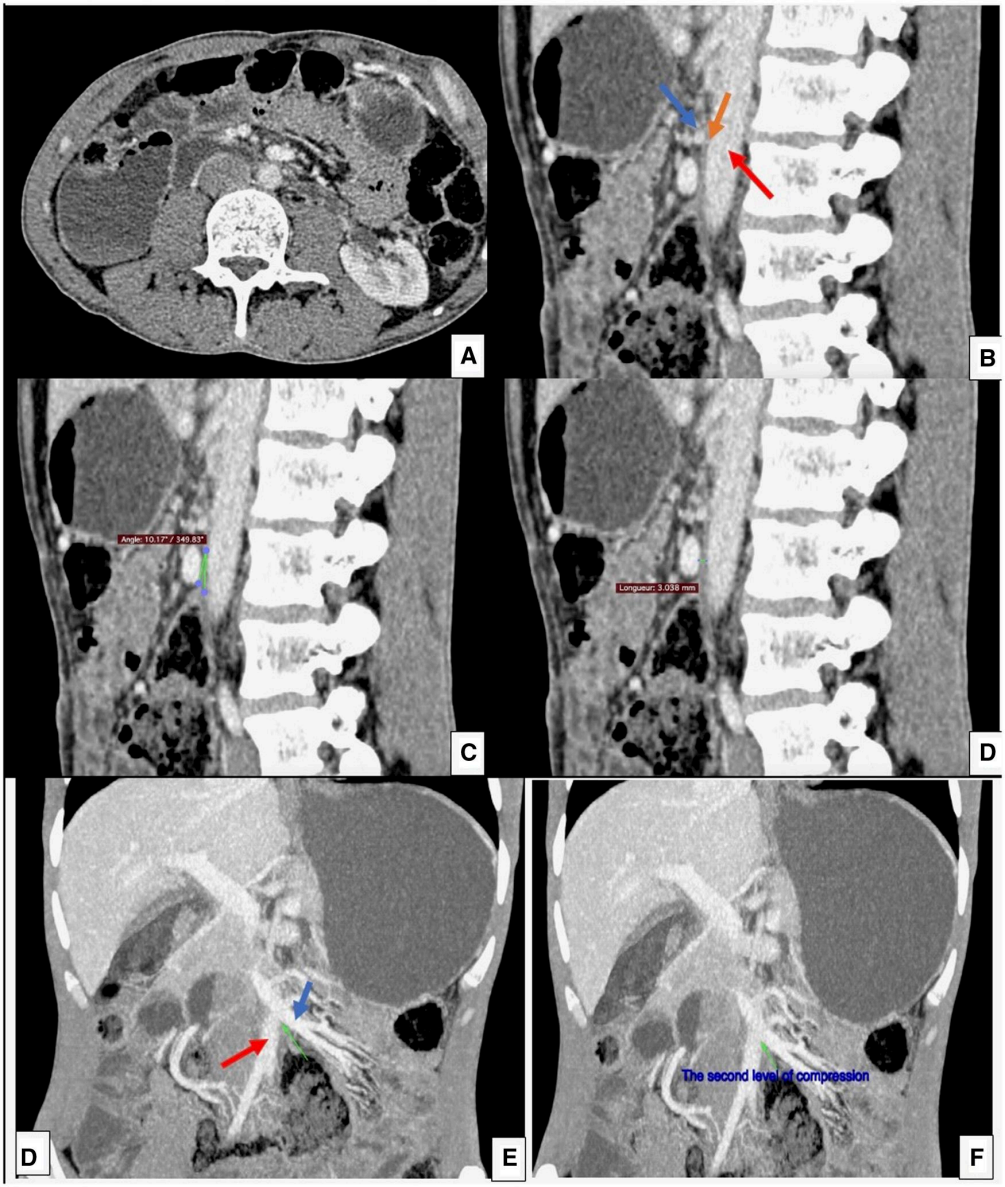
Axial (A), sagittal (B–D), and coronal (E, F) CT-enterography show the second level of compression of the third duodenal segment (middle orange arrow) between abdominal aorta (posterior red arrow) and the inferior mesenteric vein (anterior blue arrow). At this level, the compression is more marked with a narrowed angle.
